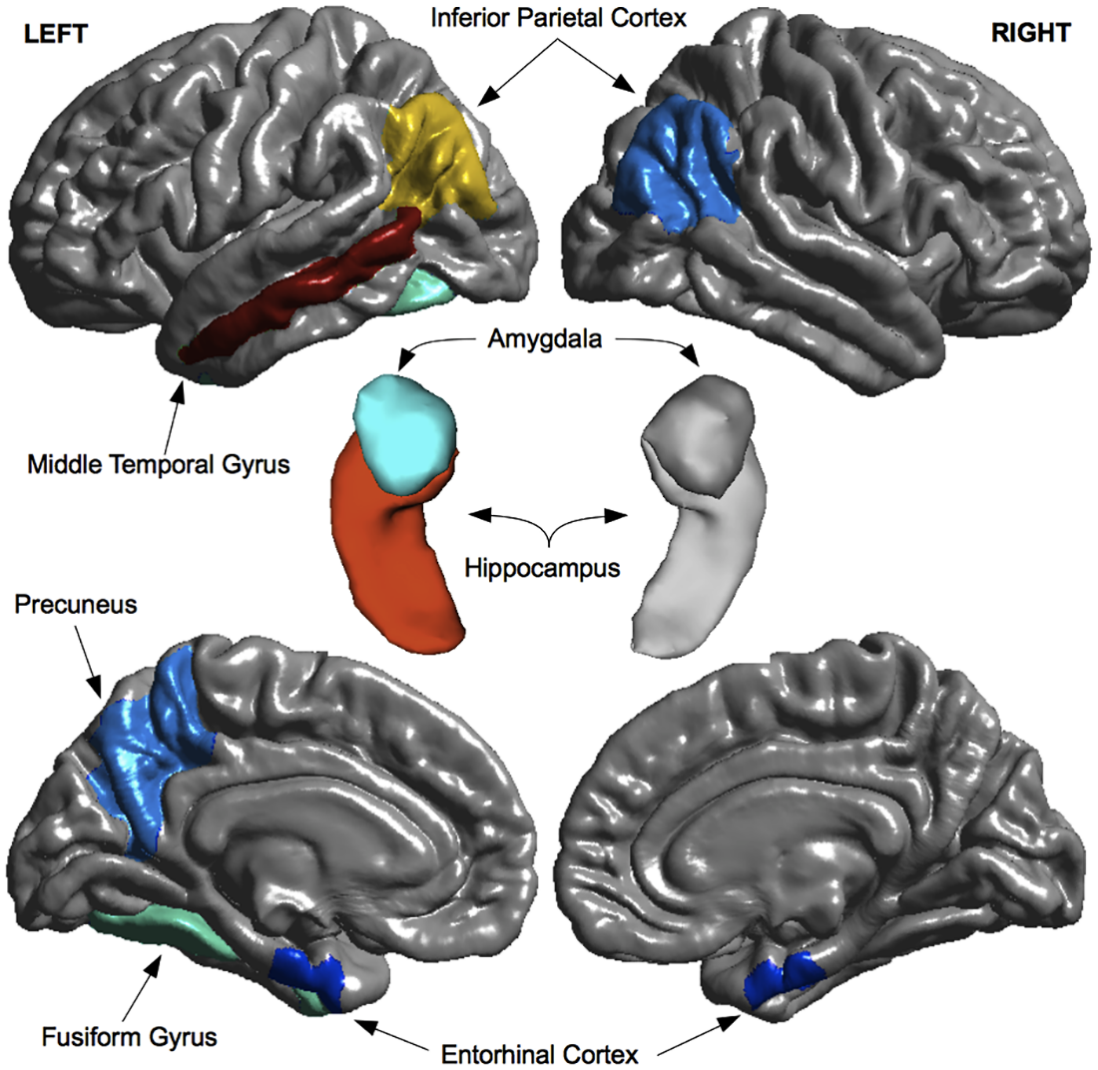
Regional MRI predictors of MCI-to-dementia progression. Morphometric measures (volumes and cortical thickness) for brain regions shown in both warm and cool colors were selected as predictors in the single-source MRI model. Morphometric measures for a subset of these regions, shown in warm colors (red, orange, yellow), were also selected as predictors in multi-source (CONCAT and MKL-Gaussian) models. Regions of interest are overlaid on top of 3-D model reconstructions of the brain (gray). Top row: lateral view of the cerebral hemispheres. Center: close-up view of the hippocampus-amygdala complex. Bottom row: medial view of the cerebral hemispheres.
